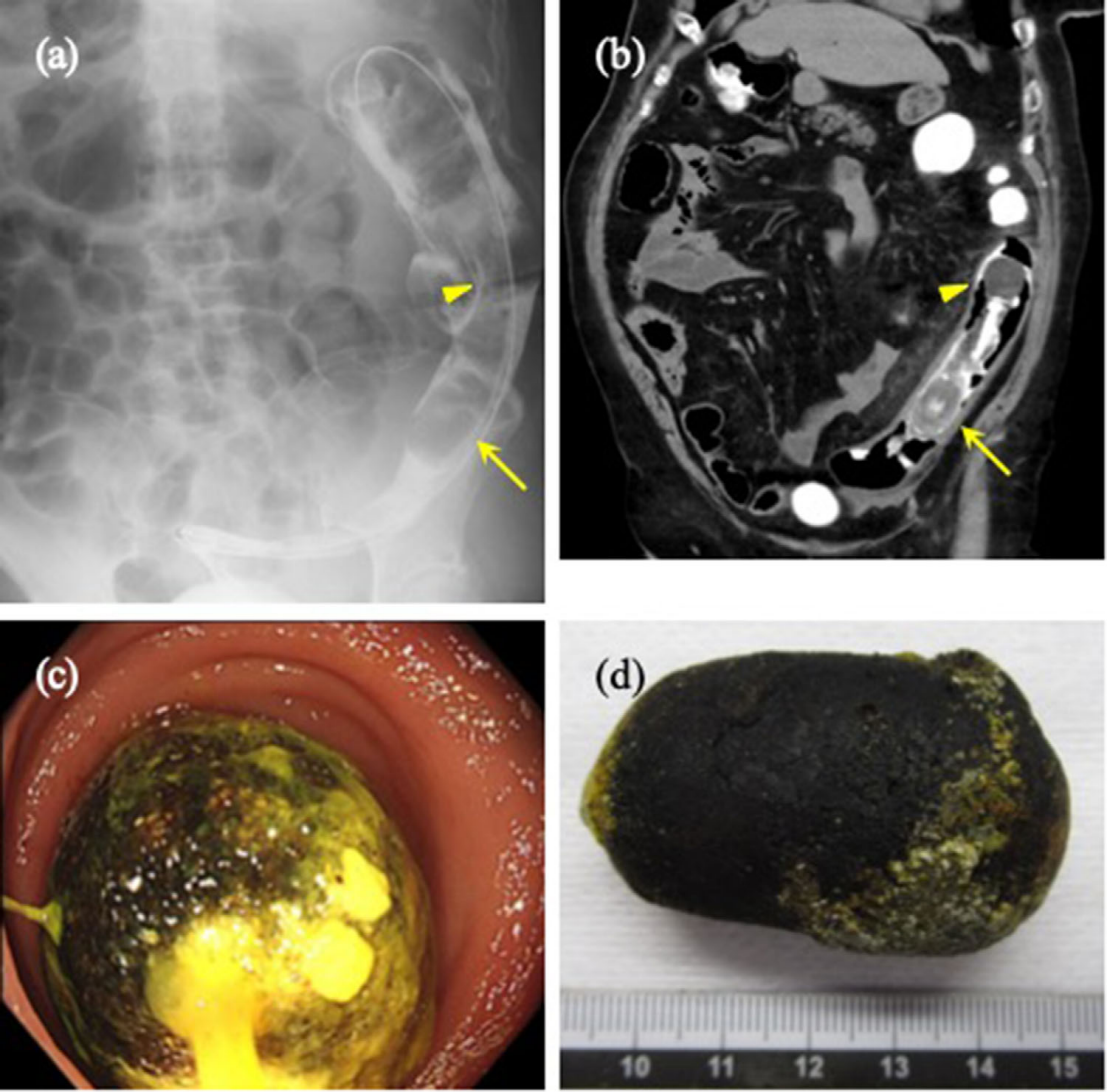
The placement of a transanal ileus tube. The gallstone (yellow arrow) and inflated balloon of the transanal ileus tube (yellow arrowhead) as seen via (a) radiography and (b) computed tomography. (c) The gallstone passed into the rectum 5 days after transanal tube placement. (d) The spontaneously dislodged and evacuated gallstone
